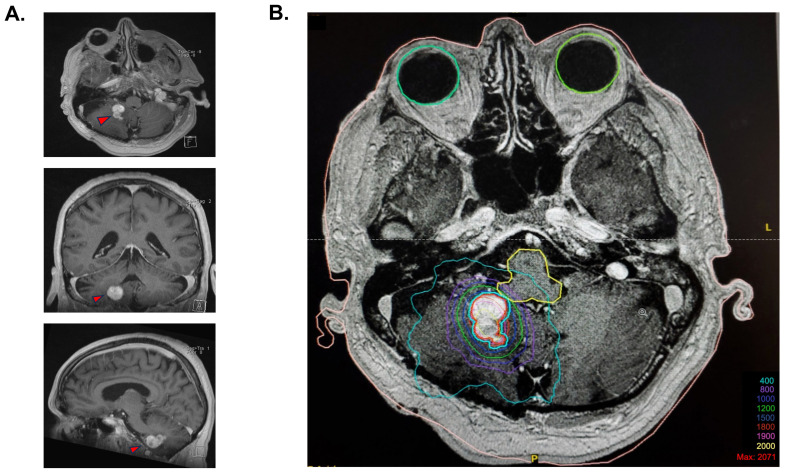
Stereotaxic radiosurgery planification. (A) Cerebral MRI T1 images prior to stereotactic radiosurgery, with a demonstration of the polylobulated lesion in the axial view, coronal view, and sagittal view. (B) Isodose coverage of the GTV (in red) for the 18 Gy radiosurgery plan. The blue contour is an expansion of 1 mm of the GTV treated at an 87% isodose because of the proximity with the brainstem. A total of 83% of the tumor volume was covered by the 18 Gy isodose, and 99.9% was covered by the 15 Gy isodose.
